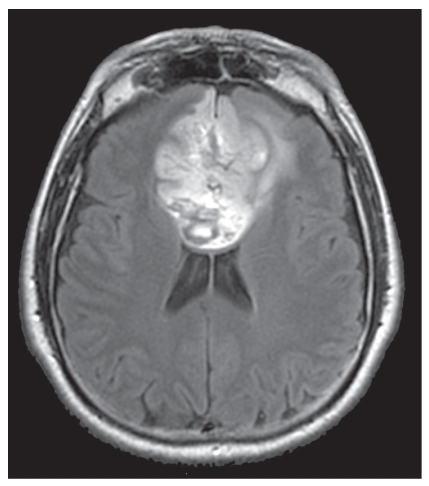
Axial FLAIR MR shows a large inhomogeneously hyperintense, extraaxial mass, located in the frontobasal region. The lesion compresses and displaces both the frontal horns of lateral ventricles and the corpus callosum.
